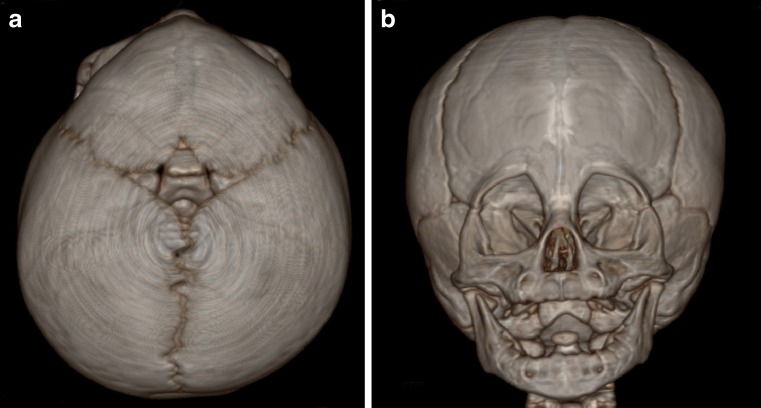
a CT scan of metopic synostosis (*top view*). b CT scan of metopic synostosis (*AP view*)
